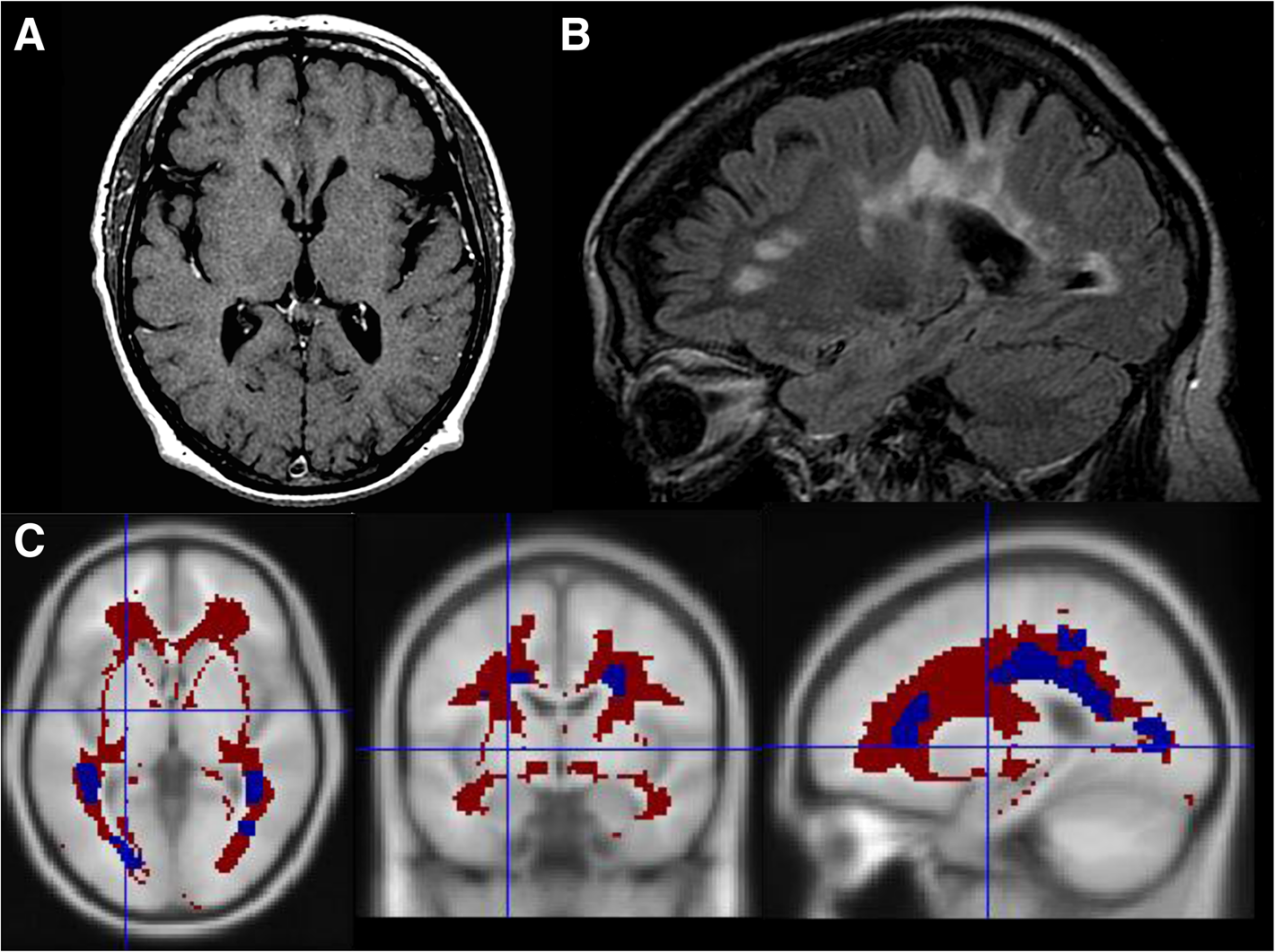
NAWM and DWM segmentation a T1-weighted sequence; b FLAIR sequence; c NAWM (red) and white matter lesion (blue) segmentation on a T1 template
